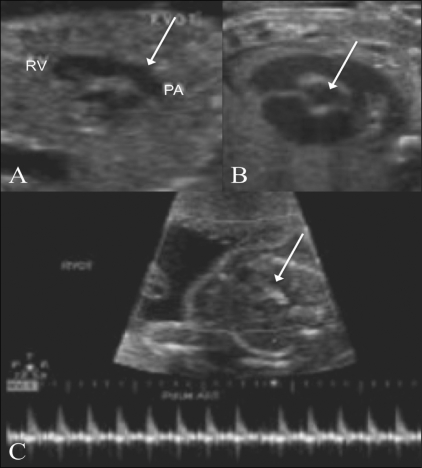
Right ventricular outflow. The pulmonary artery (PA) is seen in long axis (arrow in A) and short axis (arrow in B). Color Doppler (C) image shows color flow and the spectral waveform of the pulmonary artery (arrow). RV – right ventricle
